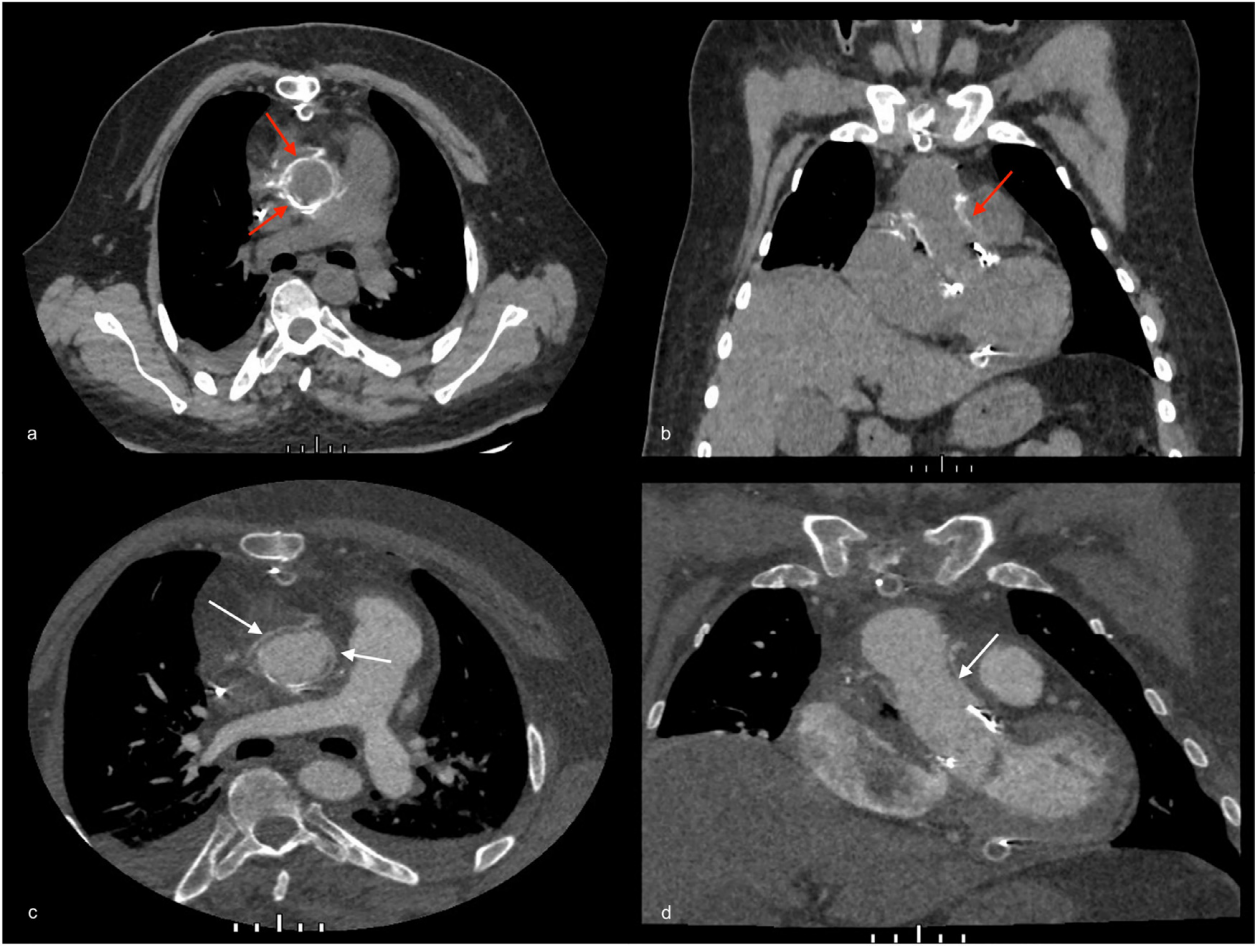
Postoperatory CT scan performed 4 days after surgical treatment. The new ascending aortic graft appears strongly hyperdense on unenhanced images (A, axial; B, coronal; red arrows) and becomes hypodense on enhanced arterial phase images (C, axial; D, coronal; white arrows). No local complications are evident.
